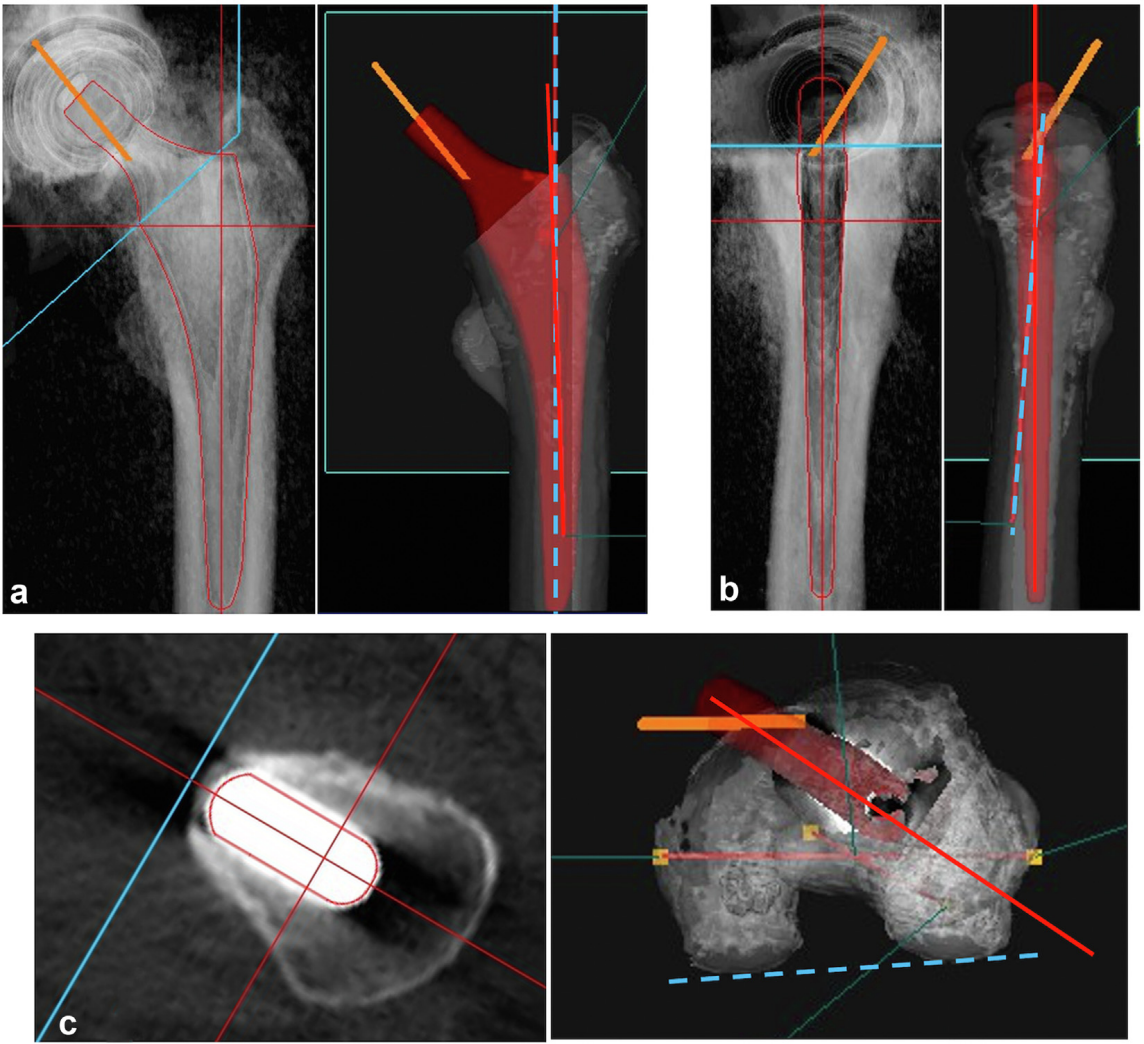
Stem alignment determination using a 3D templating software program and computed tomography. (a) Anteroposterior (AP) view for varus angle: The AP view is utilized for assessing the varus angle. (b) Lateral view for flexion angle: The lateral view is employed to evaluate the flexion angle. The blue dotted line represents the femoral axis, while the red solid line depicts the stem axis. The varus or flexion angle is derived from the angle between these 2 lines. (c) Axial view: In the axial view, the blue dotted line corresponds to the posterior condylar line (tabletop plane), and the red solid line represents the stem neck axis. The anteversion angle is calculated based on the angle between these 2 lines.
